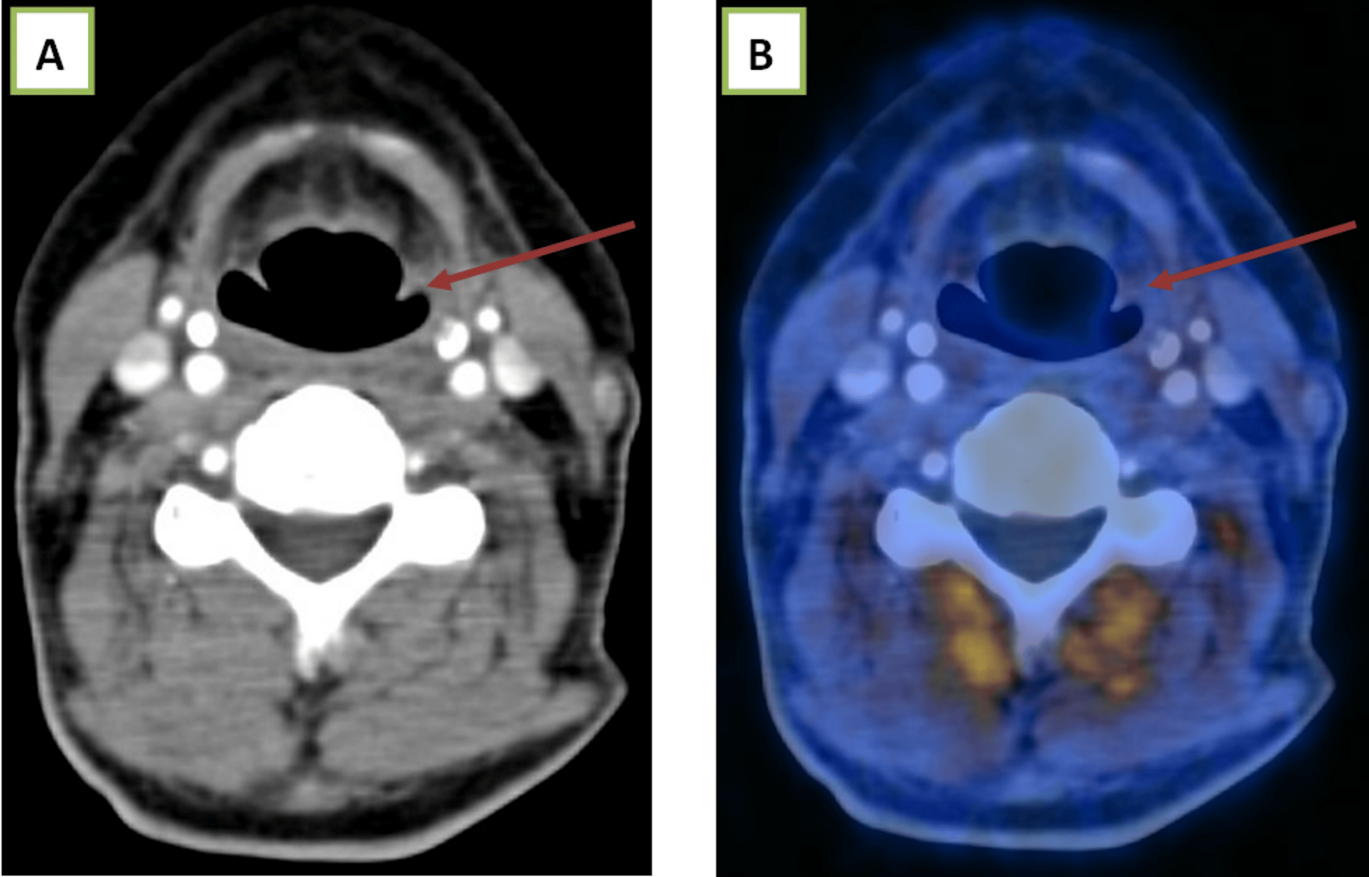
(A) Contrast-enhanced CT and (B) fused PET-CT images show mild thickening of the left aryepiglottic fold with no significant uptake on the fused images, corresponding to a Ni-RADS 1 category. PET-CT, positron emission tomography-computed tomography; Ni-RADS, Neck Imaging Reporting and Data System
